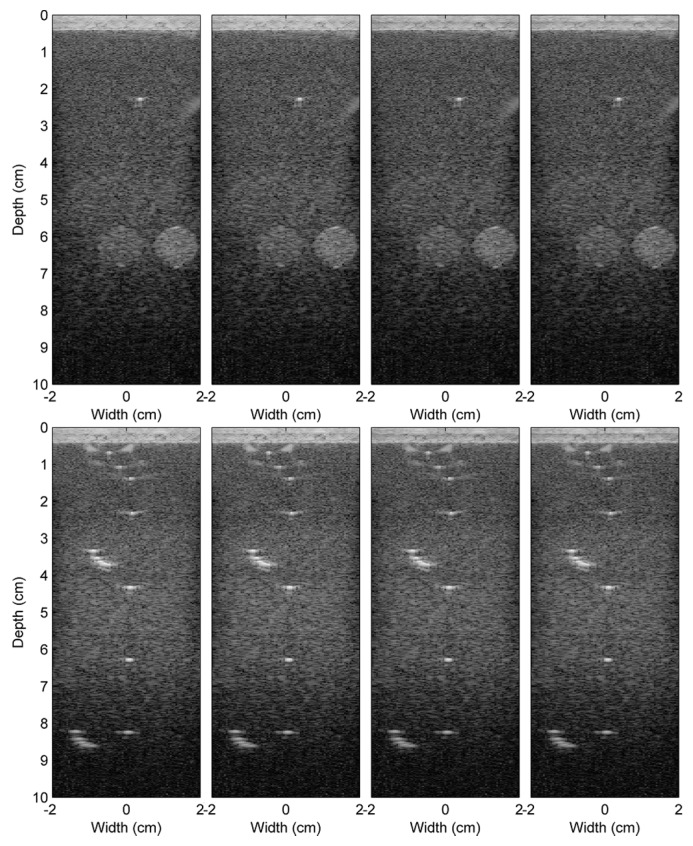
Comparison of the ultrasound images from Experiments 2 (top row) and 3 (bottom row) reconstructed usingfour4 different schemes, C-N-D, C-IQ-D, P-N-D and P-IQ-D, from the left to the right, respectively.
